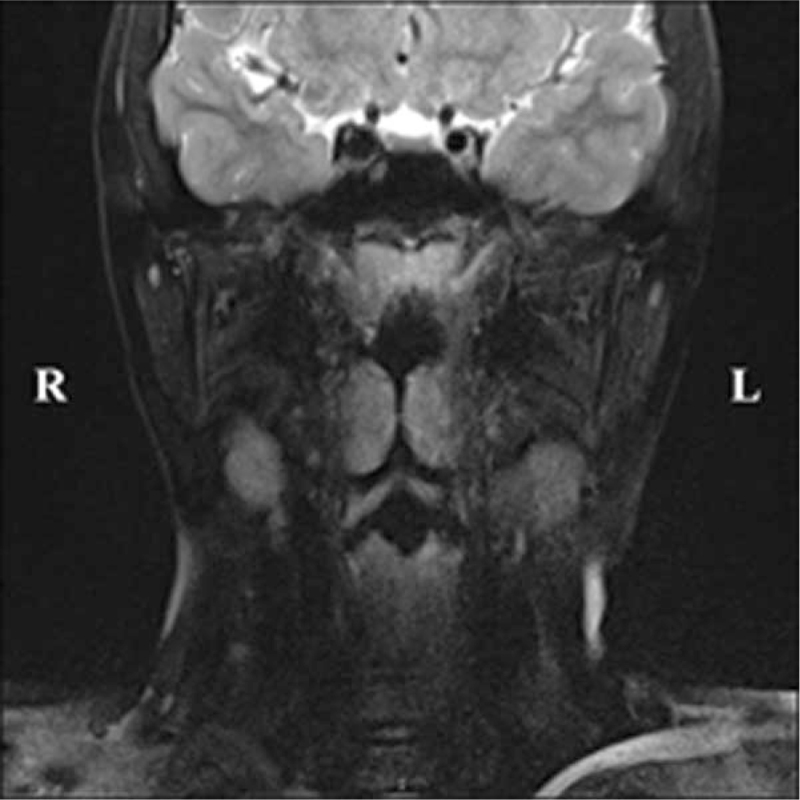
Plain MRI of the nasopharynx 6 months after the surgery demonstrates that the original cystic mass is not found. MRI = magnetic resonance imaging.
